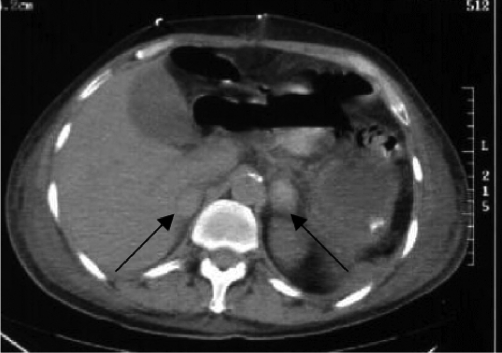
CT scan of the abdomen and pelvis. Arrows demonstrate bilateral enlargement and hyperdensity of the adrenal glands consistent with adrenal haemorrhage.
